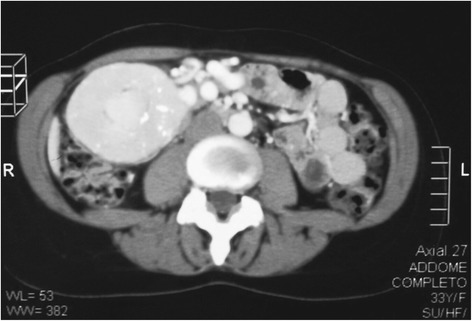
CT scan showing a solid inhomogeneous mass, with inner calcifications
